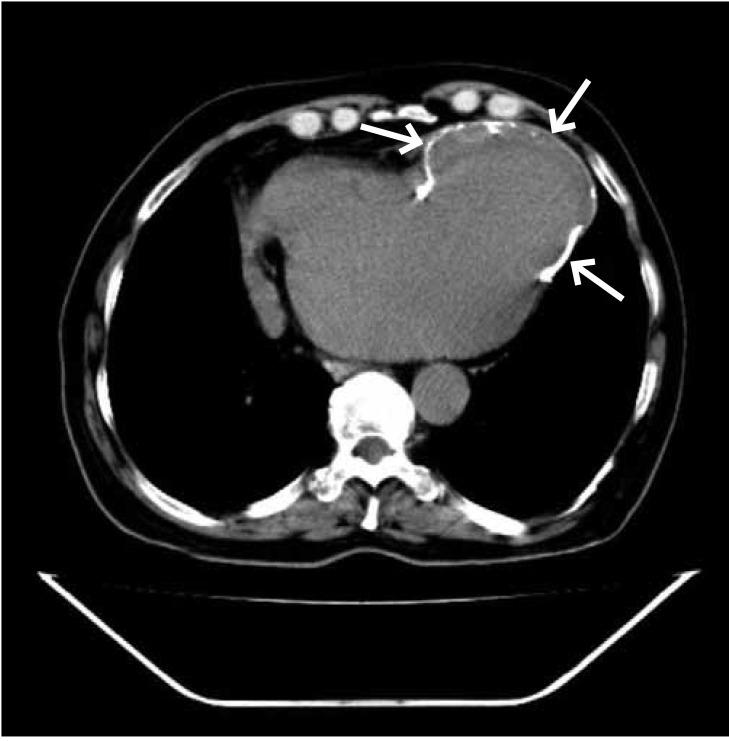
Cardiac computed tomography confirming the left ventricle aneurysm (the arrows show the edge of the calcified left ventricle aneurysm).
